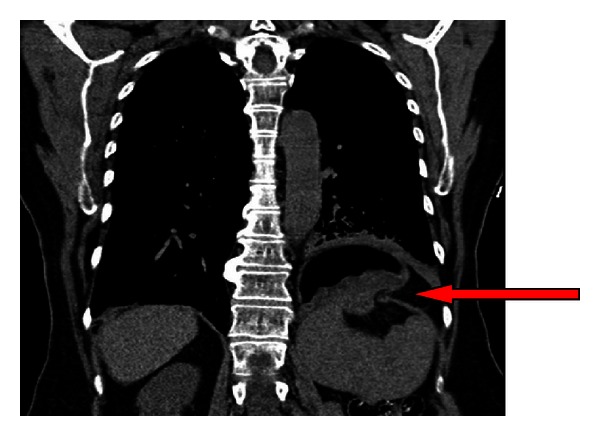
CT Case 2; coronal section demonstrating herniation of the gastric fundus through a diaphragmatic defect-note (as indicated by arrow) air-fluid level in herniated fundus indicating obstruction.
